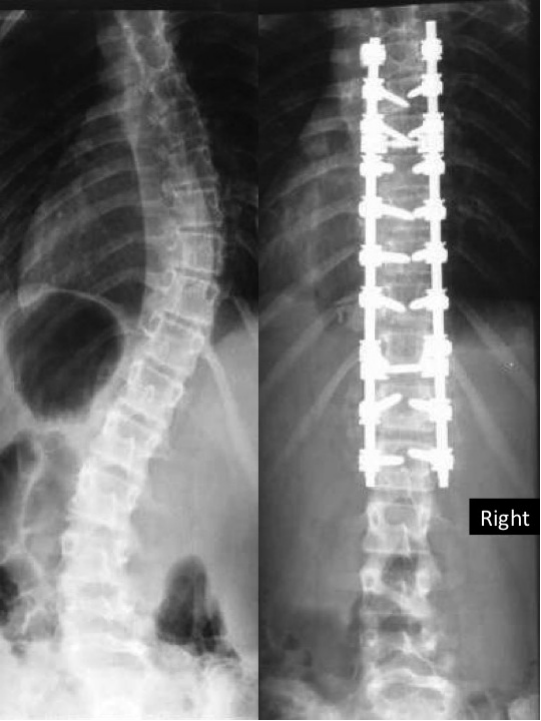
Standing postero-anterior preoperative (left) and postoperative (right) radiographic images showing correction of scoliotic deformity.
